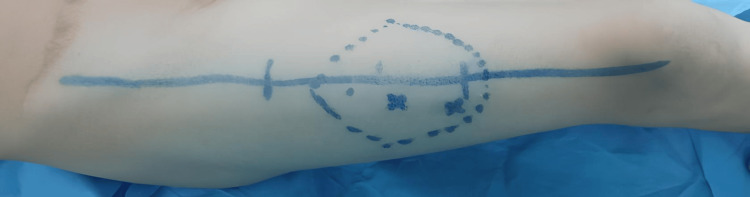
Preoperative marking of the anterolateral thigh flap. Preoperative design of the ipsilateral fasciocutaneous anterolateral thigh flap measuring 10 × 9 cm. The flap was planned along the long axis of the thigh, and the perforators identified by preoperative ultrasound mapping were marked within the skin paddle. Written informed consent was obtained from the parents/legal guardians of the patient for the publication of this case report and the accompanying clinical images.
